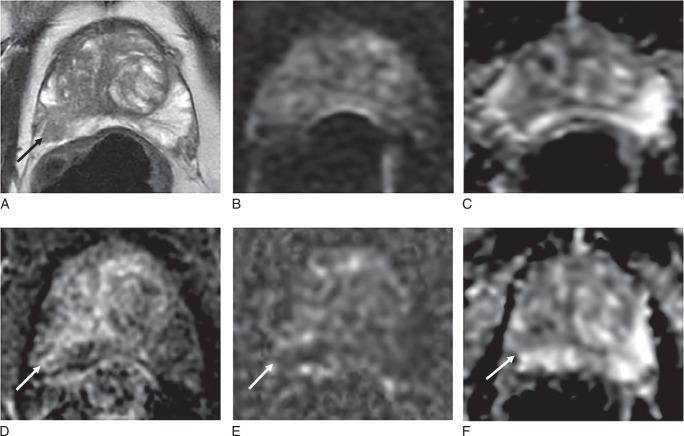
Example of a false-positive case with nondiagnostic supine DWI. Sixty-nine-year-old patient, PSA 5.17 ng/mL. A–D, Supine imaging shows ill-defined low T2 signal in the right mid PZ, PI-RADS 3 (arrow, A), poor quality DWI, PI-QUAL 1/4 (B, C), with focal early enhancement on DCE (arrow, D). E and F, Prone DWI is diagnostic and PI-RADS score 2. PI-RADS score 4 (3+1) using supine imaging alone with nondiagnostic DWI, but PI-RADS score 2 when using prone DWI.
